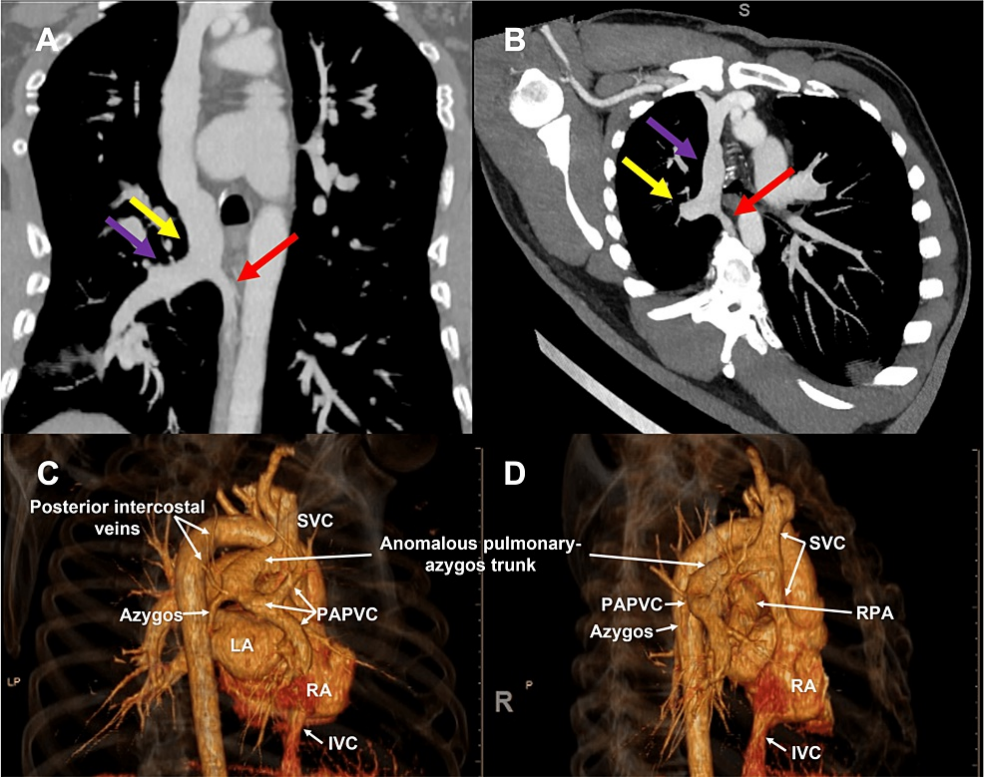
Contrast-enhanced chest CT revealing the PAPVC with oblique views (A and B) describing the junction between the anomalous right pulmonary vein (purple arrow) to the cranial aspect of the SVC (yellow arrow), as well as the enlarged azygous vein (red arrow) connecting to the anomalous pulmonary vein. Usually, the right superior pulmonary vein drains the right upper and middle lobes. The right inferior pulmonary vein drains the right lower lobe. However, this patient lacked any functional venous connection to the left atrium from the right lung. Three-dimensional CT reconstruction (C and D) demonstrates the right PAPVC to the SVC with the azygous vein and intercostal veins draining into the right anomalous pulmonary vein. RA = right atrium; IVC = inferior vena cava; SVC = superior vena cava; PAPVC = partial anomalous pulmonary venous connection
